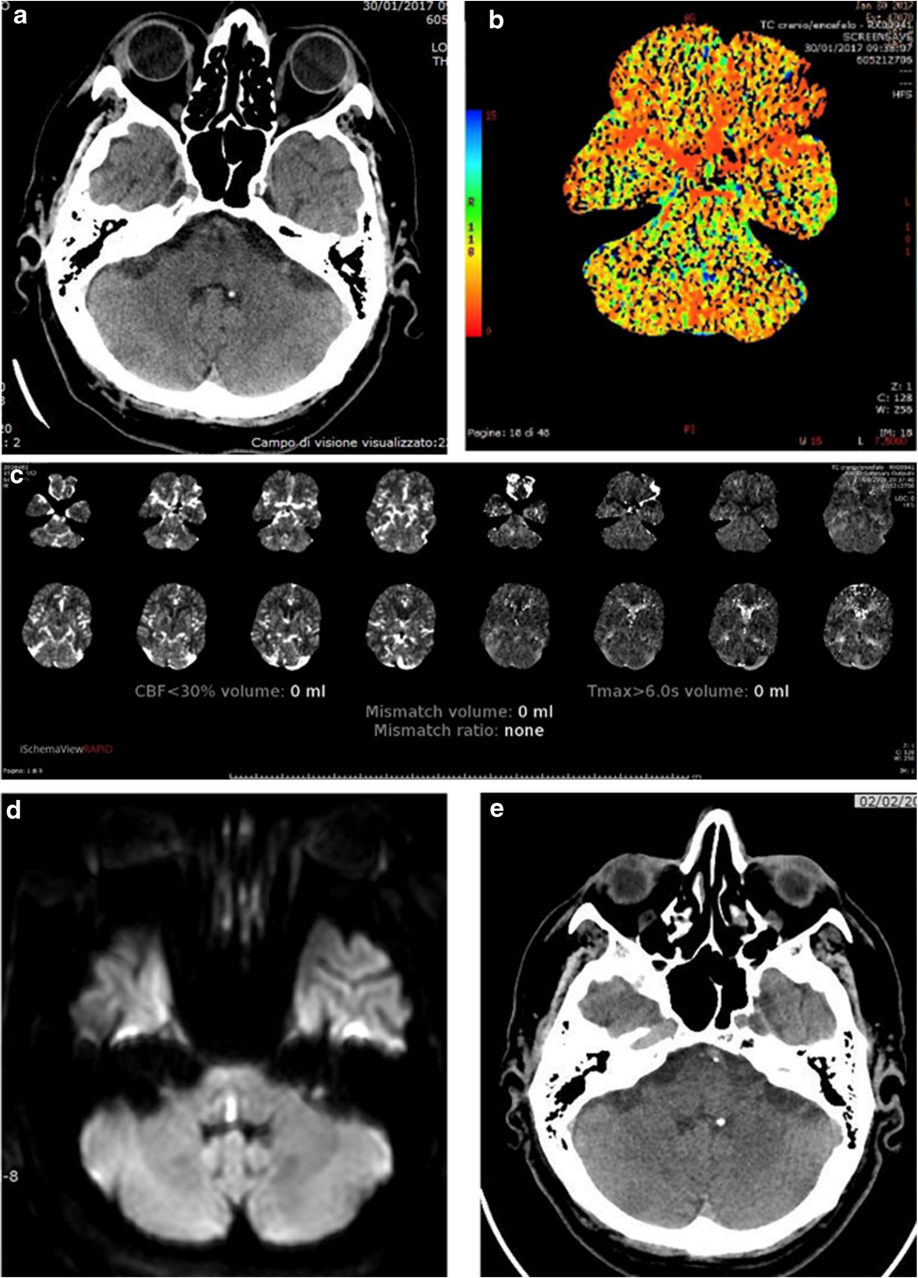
Mesencephalic lacunar infarct not clearly identified on initial NCCT (a), neither on colour-coded MTT (b) map and RAPID automatic mismatch map (c) but detected by DWI (d) and confirmed on follow-up CT (e)
